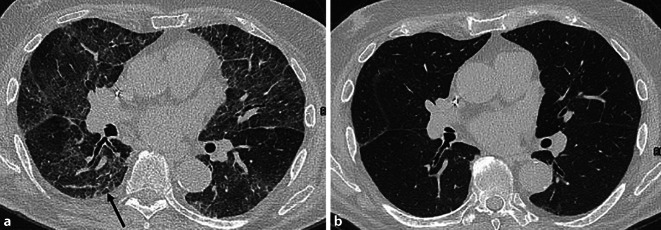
CT-Thorax eines männlichen Patienten mit bekannter rheumatoider Arthritis und kürzlich initiierter Methotrexat-Therapie, der sich notfallmäßig mit progredienter Dyspnoe und Husten vorstellte. a Ausgedehnte peribronchovaskulär und subpleural betonte Ground-Glass-Opazitäten, akzentuierte interlobuläre Septen sowie subpleurale Retikulationen (*schwarzer Pfeil*). Das radiologische Muster wurde als Methotrexat-induzierte Pneumonitis im Sinne eines fibrosierenden Musters nichtspezifischen interstitiellen Pneumonie (NSIP) interpretiert, woraufhin die Methotrexat-Therapie umgehend abgesetzt wurde. b Kontrolluntersuchung 4 Wochen später: vollständige Rückbildung der pulmonalen Veränderungen – mit sowohl diagnostischem als auch therapeutischem Aussagewert
